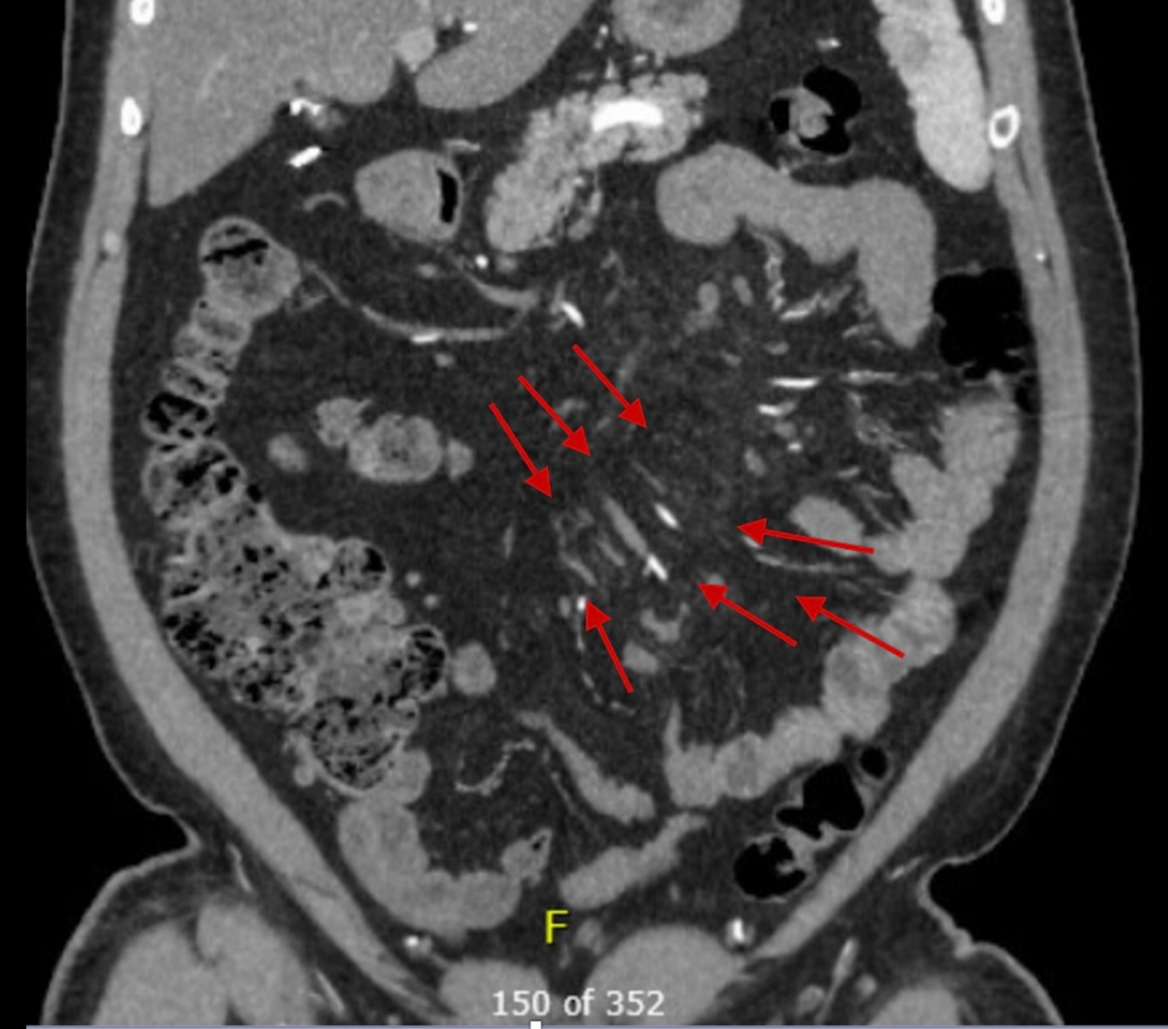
Computed Tomography of the Abdomen The red arrows denote the areas of mesenteric fat stranding.
